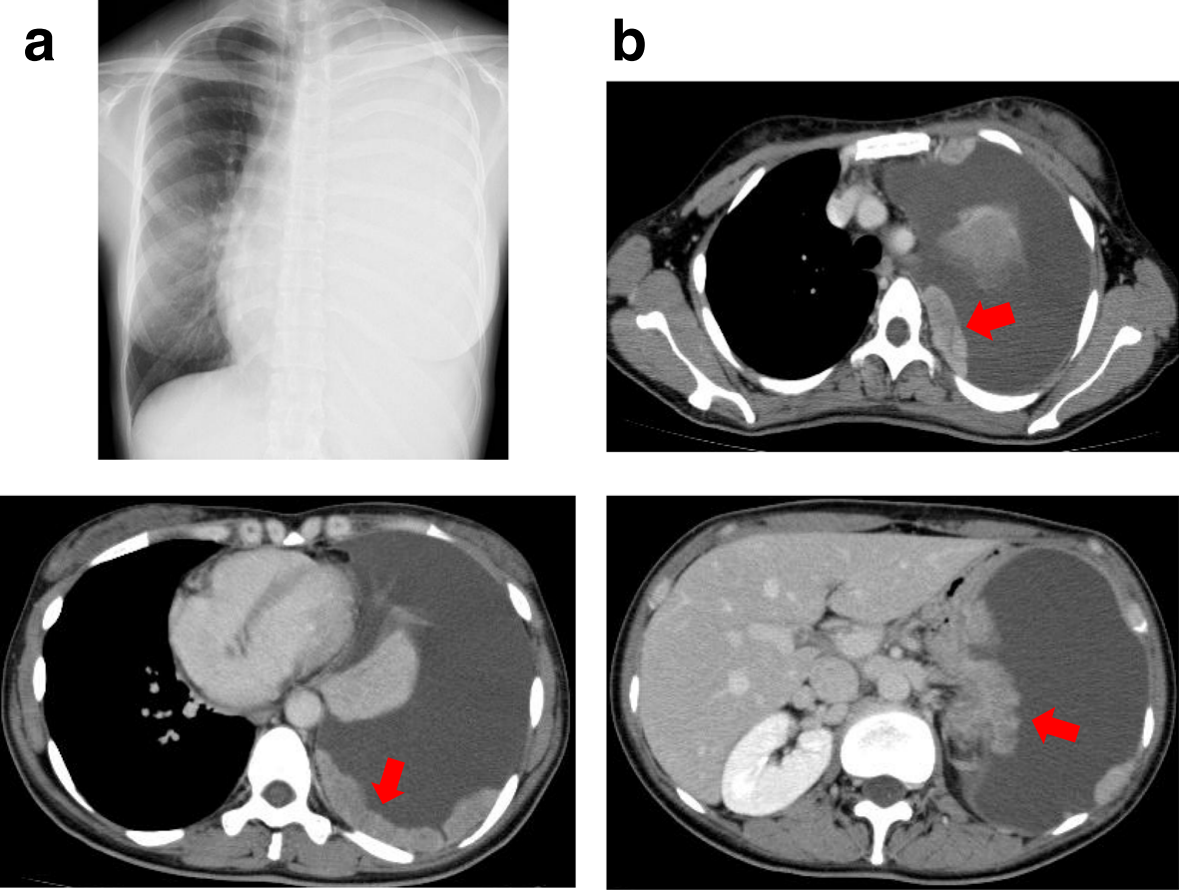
Chest X-ray and Contrast-enhanced computed tomography at the initial diagnosis. a Chest x-ray showed a large amount of pleural effusion. b Contrast-enhanced computed tomography of the chest-mediastinal window showed multiple irregular pleural thickening (the red arrows) of the left lung with pleural effusion
